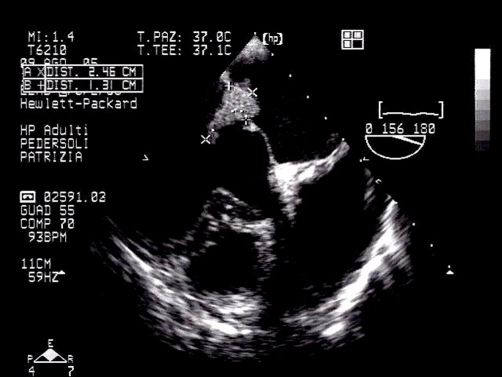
Transoesophageal echocardiography with the evidence of large vegetation attached to the atrial side of the anterior mitral leaflet.
